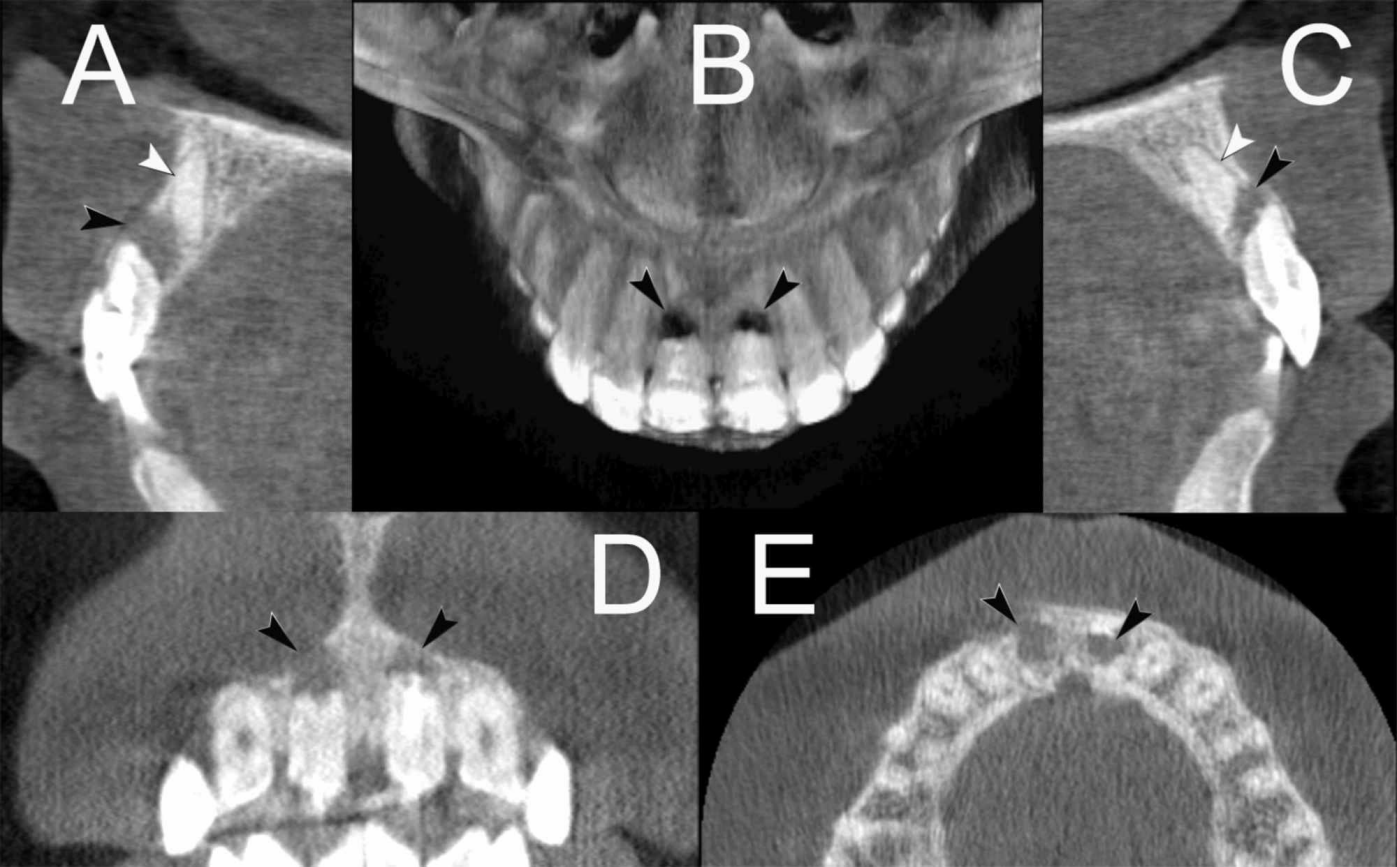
CBCT of the traumatised maxillary central incisors (A & C) through the axial section of the maxillary central incisors revealed oblique root fractures of the middle third, associated with dramatic displacement of the apical fragments. Large radiolucent lesions between the apical and coronal fragments can be seen. Horizontal root fractures at their mid-root level with a large dislocation of apical fragments with large radiolucent lesions between the coronal and apical fragments (A, B, C & E), and short coronal segments with wide-open apices (D) can be seen (White arrow head = apical segment, black arrow head = lesion). CBCT: Cone beam computed tomography
